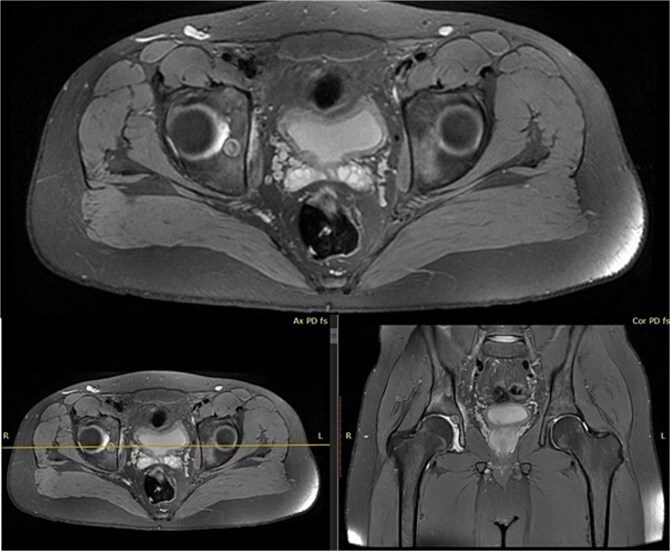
MRI findings supporting initial CT diagnosis.
